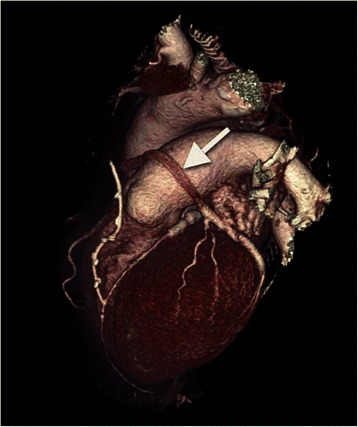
Coronary Computed Tomographic Angiography of a Partially Occluded Meshed SVG cCTA three-dimensional reconstruction showing a partially occluded eSVS® supported SVG between its proximal aortic and first distal sequential anastomosis (arrow). However, a contrast filling of the distal SVG between the first and last distal sequential anastomosis confirms patency in this section of the SVG
